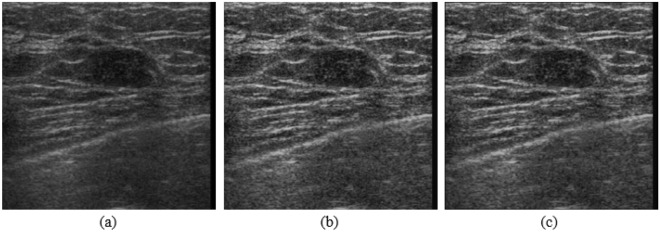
(a) Original ultrasound image; (b) image after CLAHE; (c) image after anisotropic diffusion.
